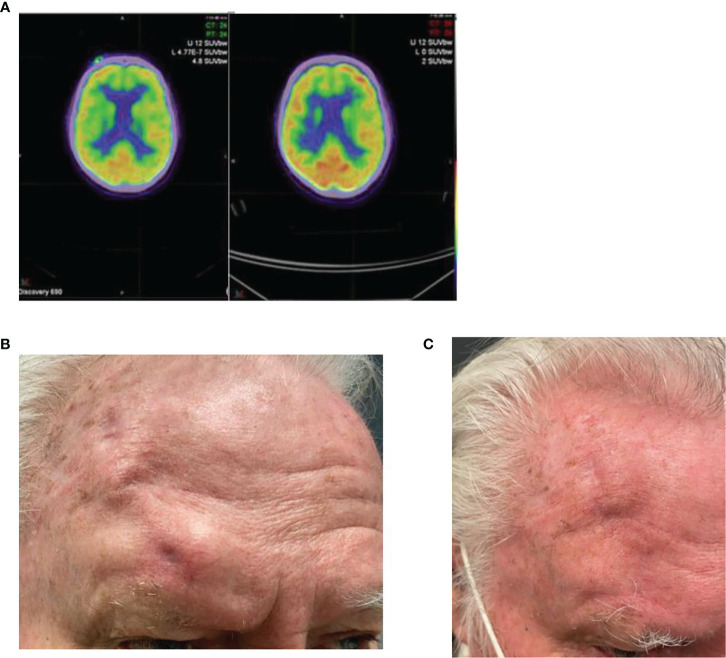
(A) Baseline FDG-PET imaging demonstrating an avid right supraorbital cutaneous malignancy with a complete metabolic response seen on follow up FDG-PET post four cycles of cemiplimab 350 mg/3-weekly. (B) Baseline photo of the right supraorbital clinical mass. (C) Post 7 cycles of cemiplimab demonstrating complete resolution.
